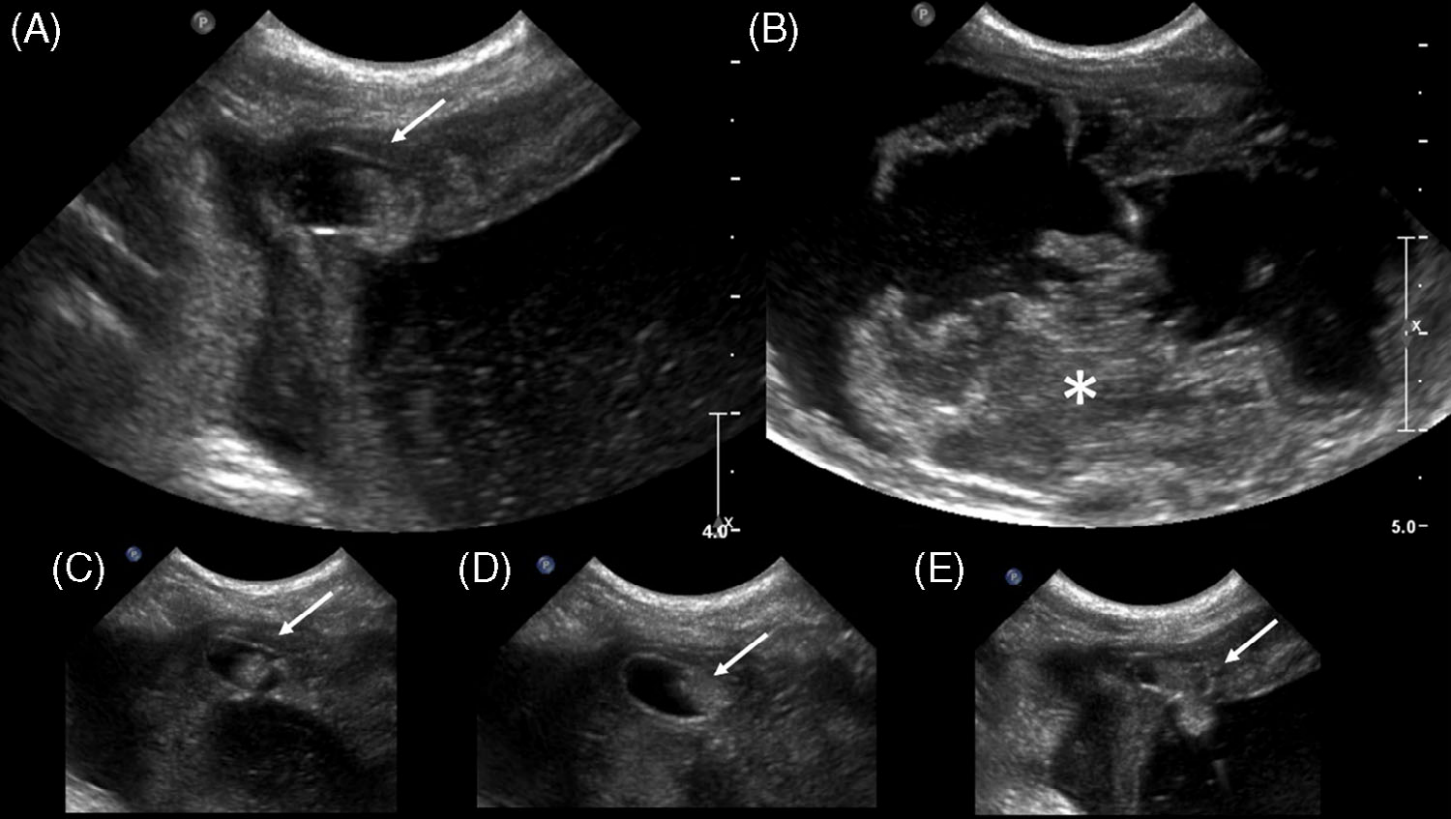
Longitudinal (A and B) and transverse (C–E) ultrasound images of the urinary bladder. An ovoid cavity approximately 1 cm mediolateral by 0.5 cm dorsoventral and craniocaudal is identified at the urinary bladder apex (arrow) which contains a combination of anechoic fluid and echogenic material. This cavity communicates with the bladder lumen and upon light ballottement of the bladder.
